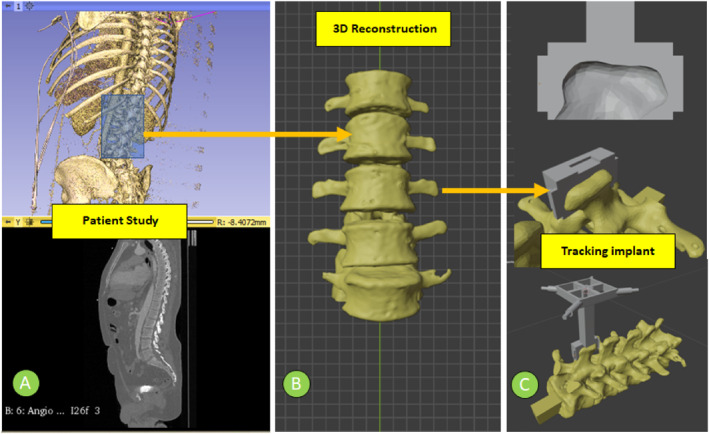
The construction process of the patient model. (A) Patient CT dataset segmentation and visualisation. (B) Three‐dimensional reconstruction of the lumbar spine. (C) Tracking implant design anatomically coupled to the spinal apophysis of the L1 Lumbar vertebra.
